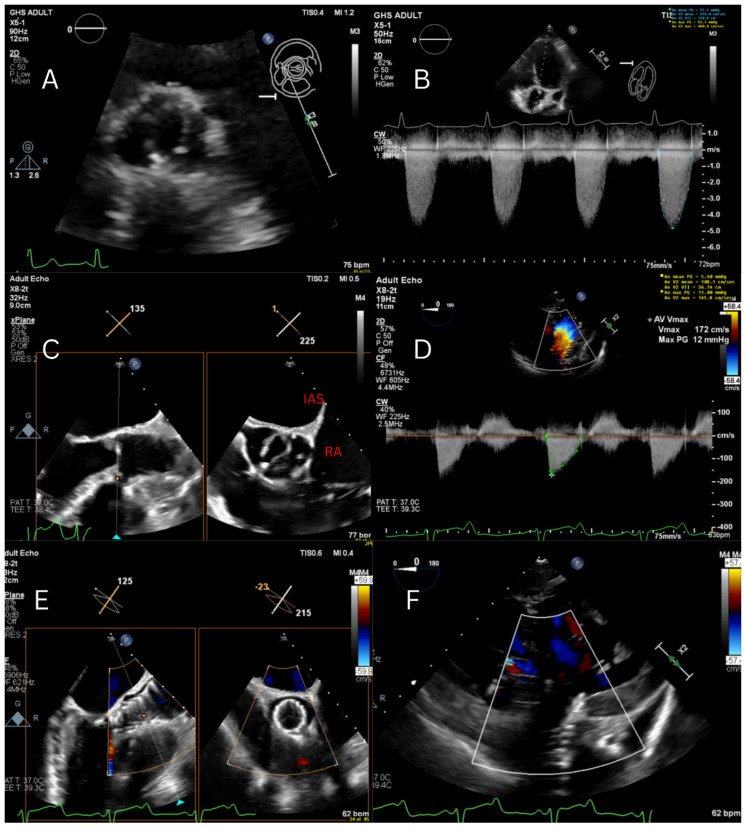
(A) Short axis view of the aortic valve on TTE showing bicuspid AV with fusion of the RCC and the NCC. (B) Aortic stenosis assessment by Doppler showing severe AS: Vmax 4.8 m/s, MG 55 mmHg, AVA 0.7 cm^2^. (C) TEE showing bicuspid AV with fusion of the RCC and the NCC (image on the right is inverted). (D,E) Immediately post-TAVR TEE result: AV MG 5 mmHg, DI 0.78, No AR. (F) Transgastric view showing no significant central or paravalvular aortic regurgitation.
